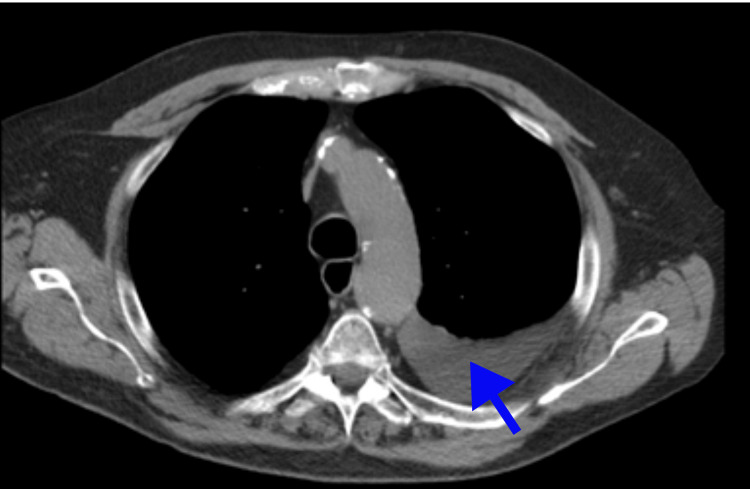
CT chest showing left-sided pleural effusion and no evidence of significant adenopathy or other soft tissue masses
